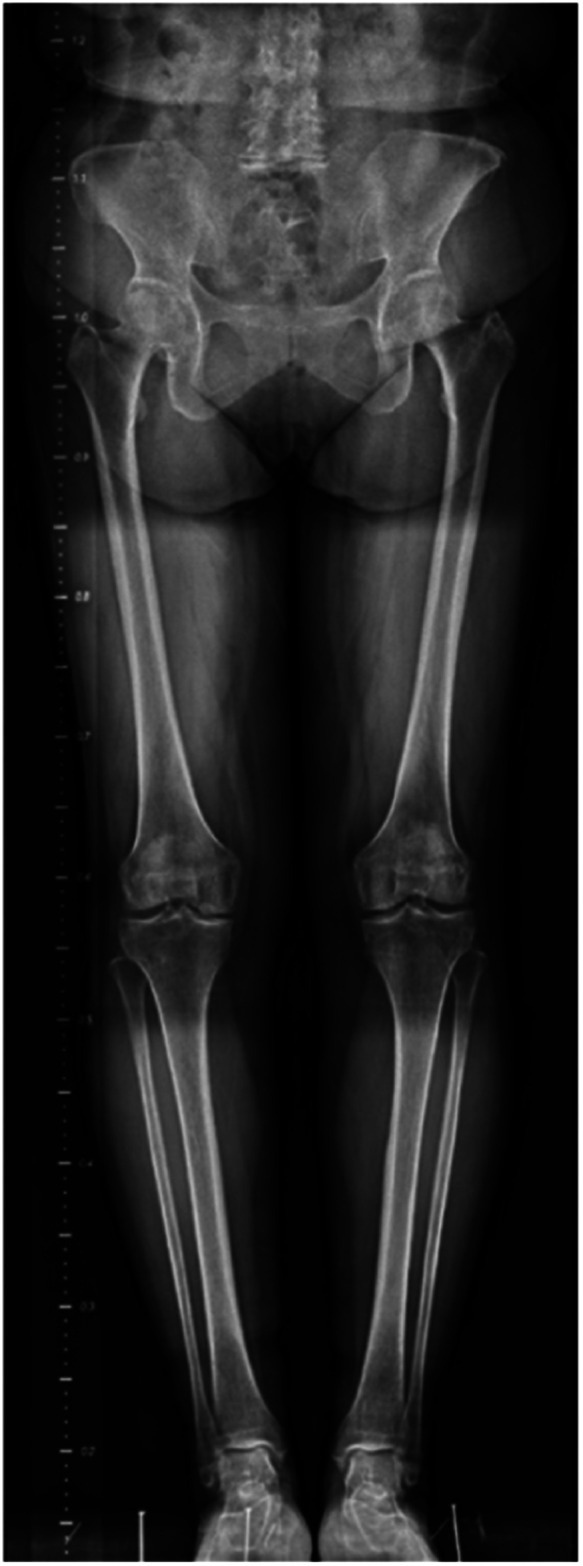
A full-length lower limb x-ray radiograph stitched using the proposed method based on canny algorithm.
